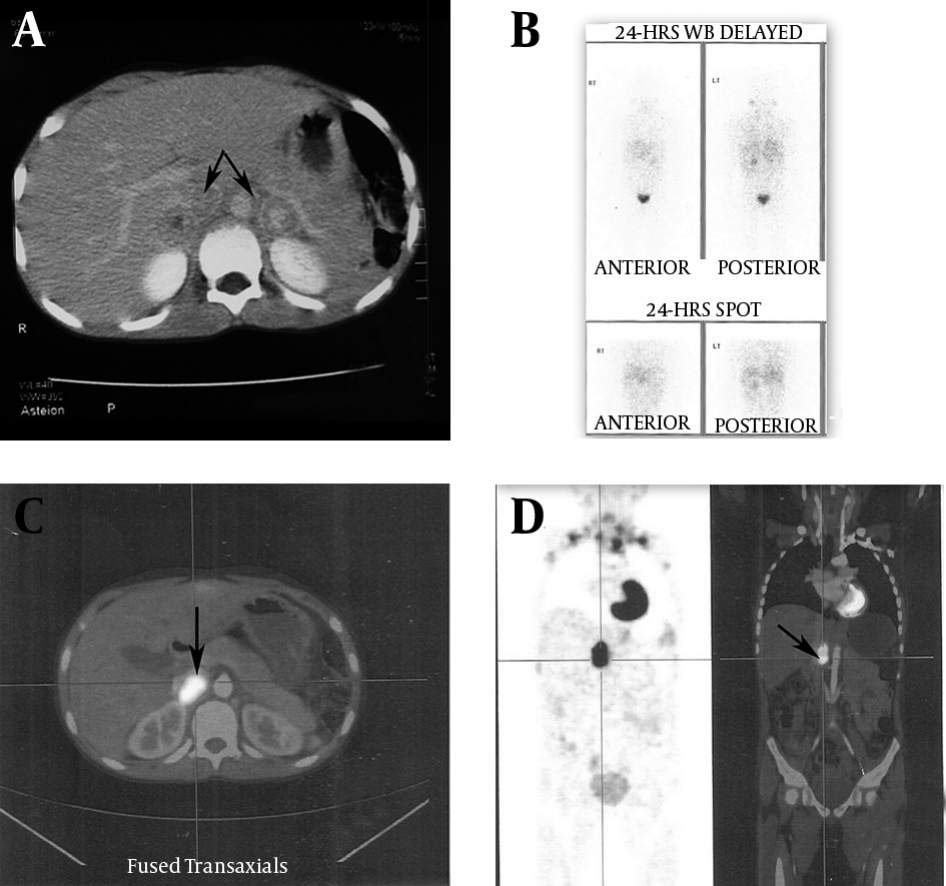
A: CT Scan of Abdomen Revealed Bilateral Pheochromocytoma, B: [^123^] I-Labelled MIBG Scan Showing Inconclusive Findings, C and D: PET Scan Revealed Metabolically Active Enhancing Nodule in the Right Suprarenal Region
